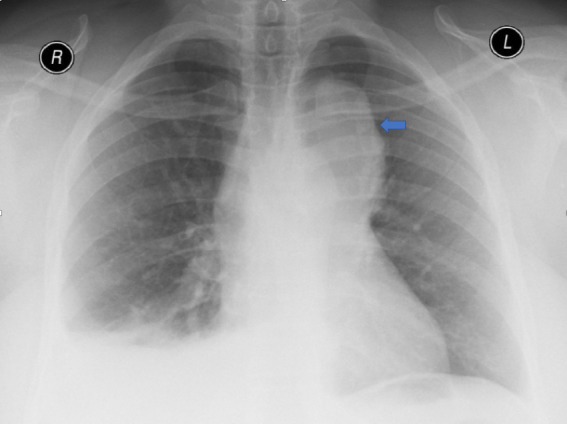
The chest radiograph, taken 4 years prior to the current
